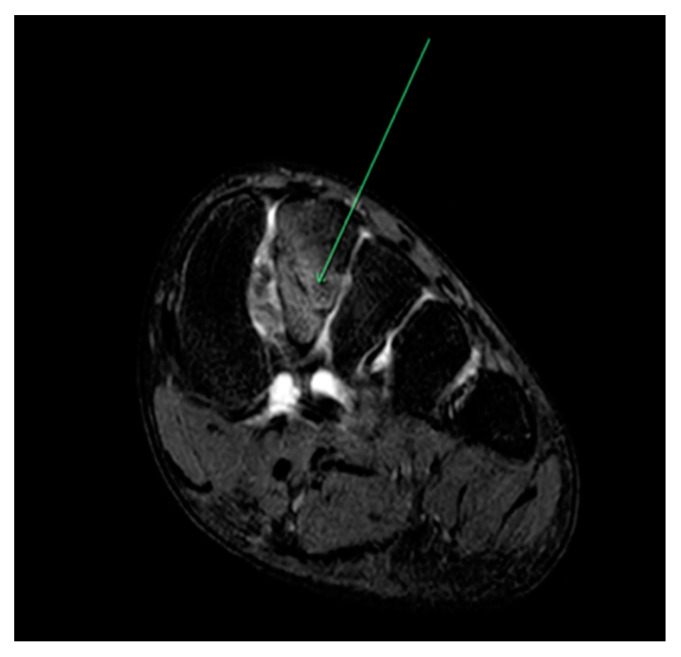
Coronal plane MRI of T2 weighted image of 2nd metatarsal stress fracture (as indicated by the green arrow) in 14-year-old ballet student training 25–30 h per week.
